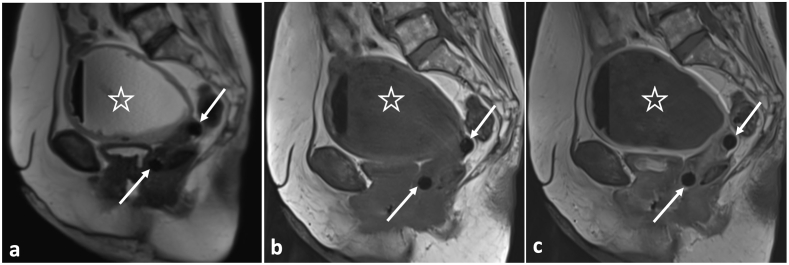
sagittal MR images. a; T2WI, b; pre-contrast T1WI. c; post-contrast T1WI. Thick-walled cystic lesion (star) containing air-fluid level and contrast enhancement in the wall. The ring pessary (thin arrows).
